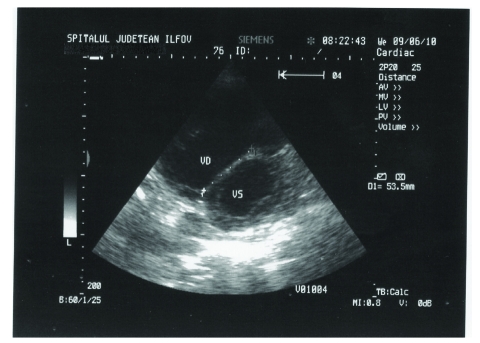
2D Transthoracic echocardiography. The flattening of the interventricular septum in parasternal short–axis view. VS=left ventricle, VD=right ventricle.
